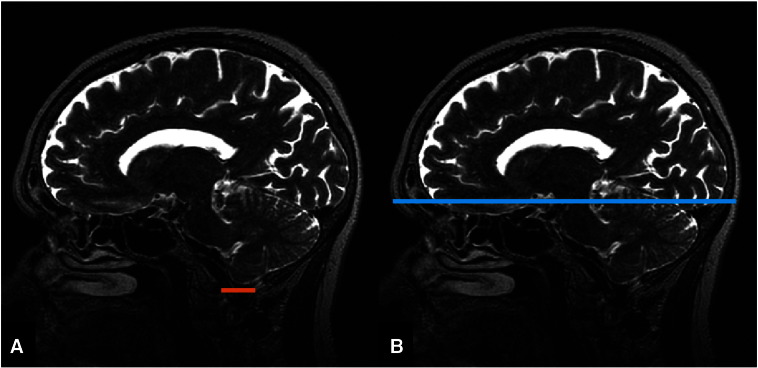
(A) sagittal slice from a T2W MRI sequence showing the inferior limit of the intracranial volume at the foramen magnum (orange). (B) identification of the inferior boundary using the upper edge of the supraorbital ridge (blue).
